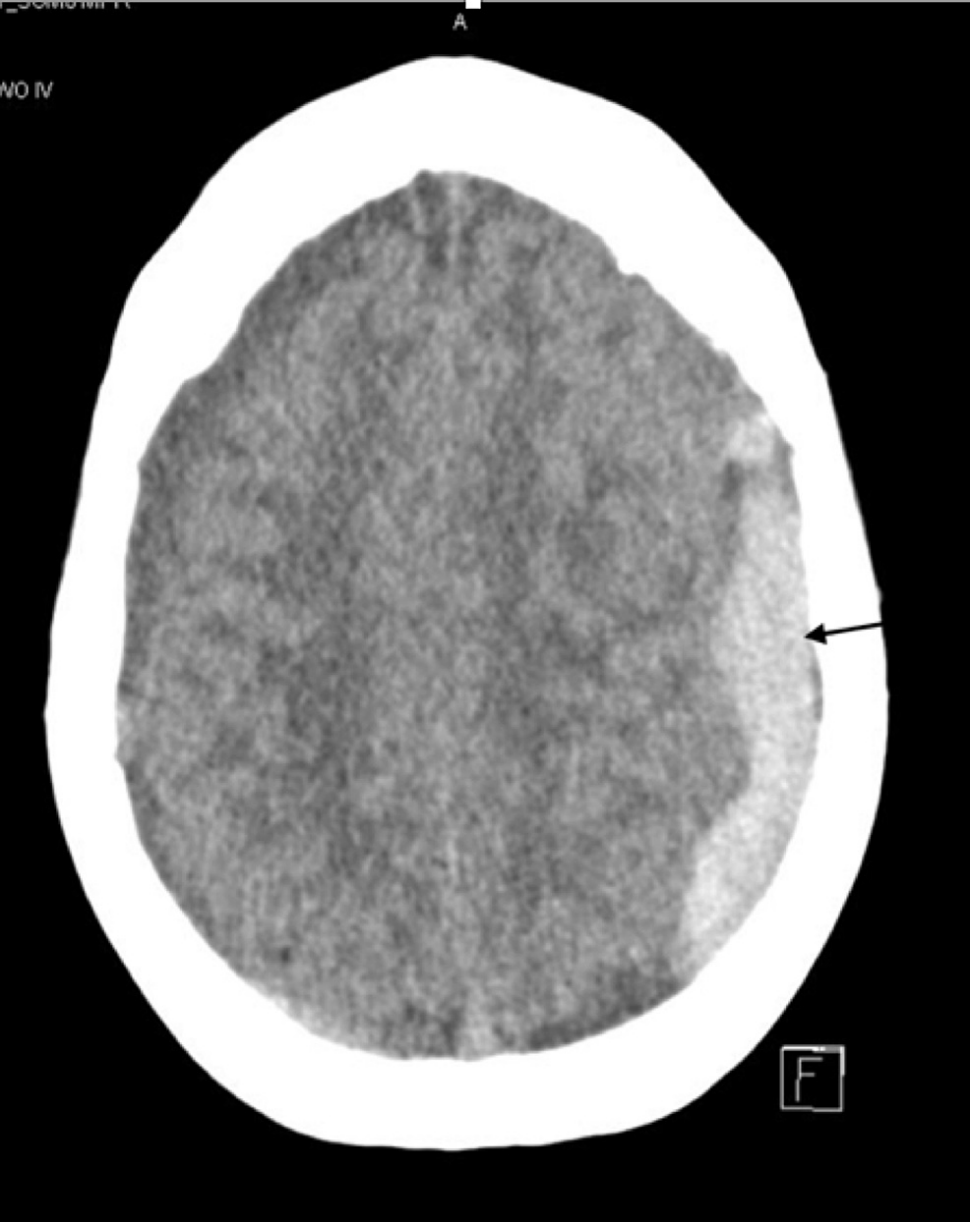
Brain computed tomography. Longitudinal section: left subdural hematoma.
